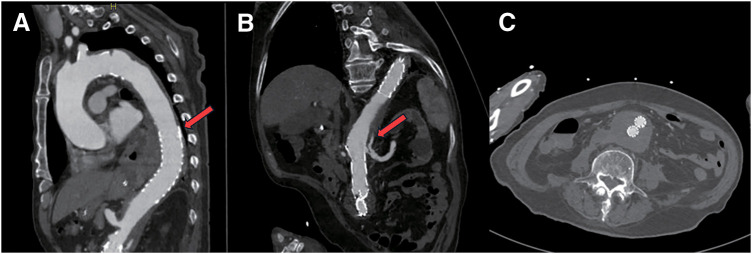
Fig. 3 Postoperative CT demonstrating false-lumen thrombosis. (A) Postoperative contrast-enhanced CT demonstrated successful TEVAR with complete closure of the primary thoracic entry tear (arrow). (B) Although a re-entry tear at the level of the renal artery persisted (arrow), only minimal residual contrast enhancement within the false lumen was observed, indicating progressive thrombosis. (C) The infrarenal abdominal aortic aneurysm had decreased in size, and the retroperitoneal hematoma showed signs of resolution. CT: computed tomography; TEVAR: thoracic endovascular aortic repair
